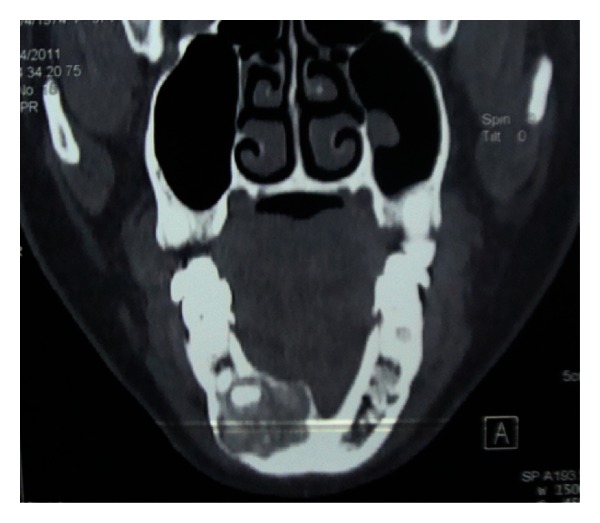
Axial CT image showing the lesion causing thinning and expansion of buccal as well as lingual cortical plate.
